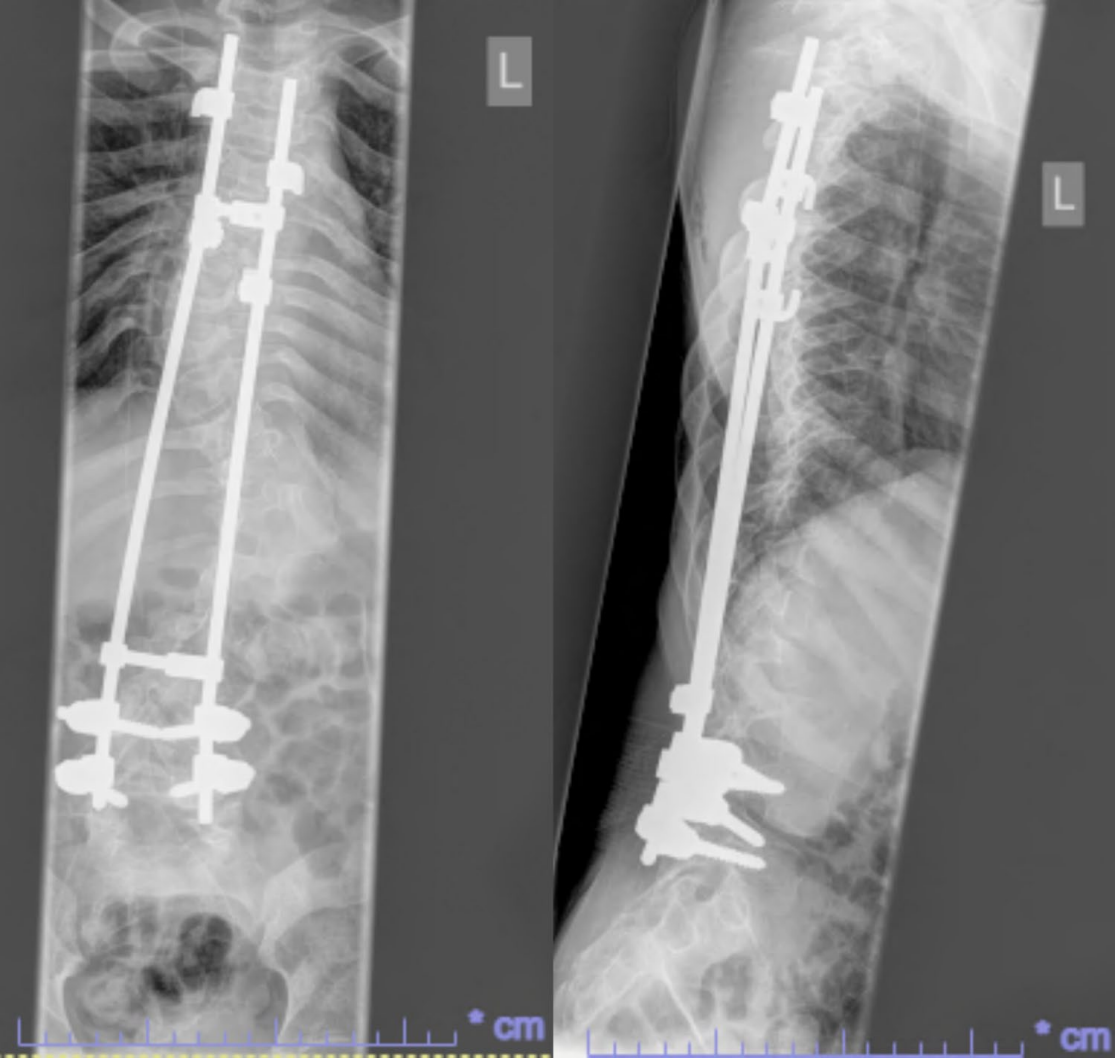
Post operative X-ray after second lengthening of TGR, aged 8
